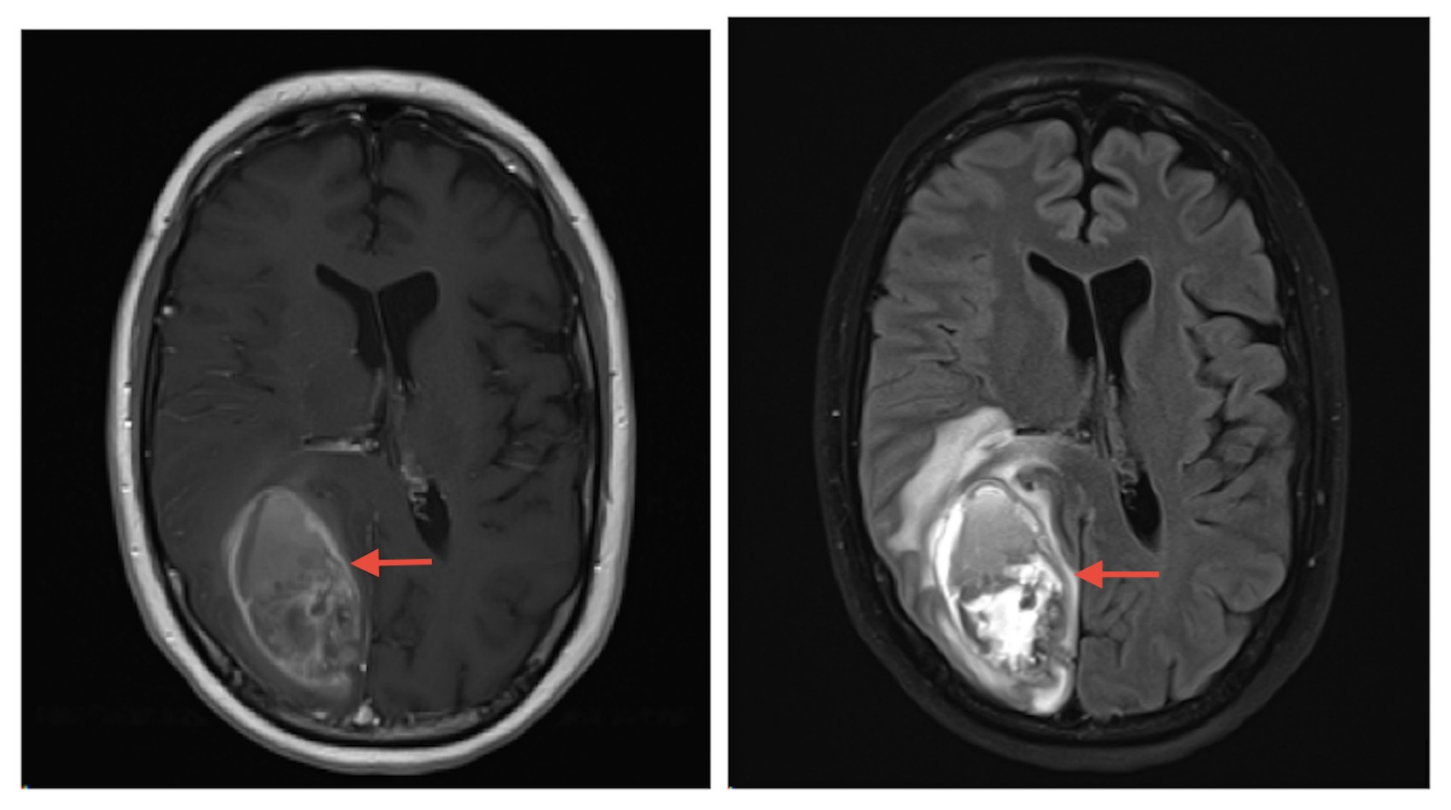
Preoperative magnetic resonance imaging demonstrating post-contrast T1-weighted (left) and T2 fluid attenuated inversion recovery (right) images of 6.3 x 3.5 x 5.3 centimeter hemorrhagic lesion in the right occipital lobe.
